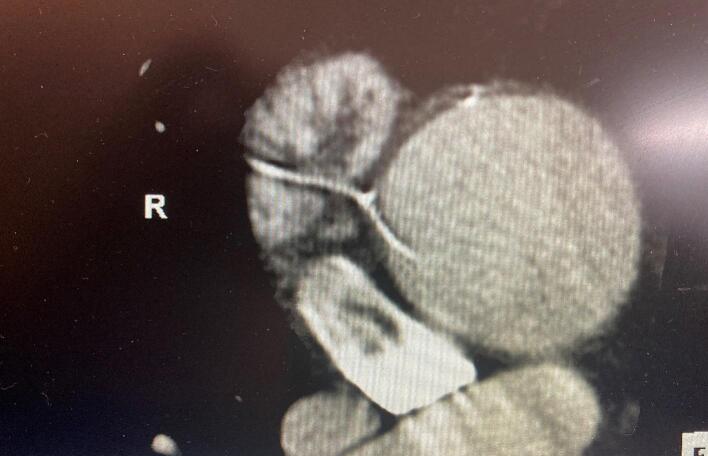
CT Imaging of Intra-Aortic TEPW.
